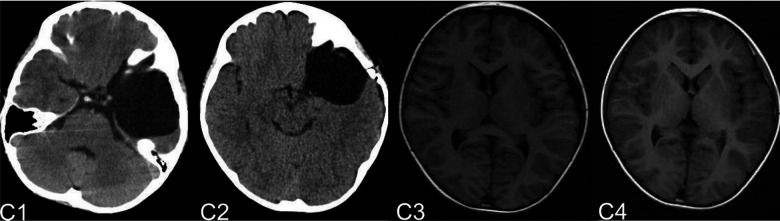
(C) shows the patient, who had subdural effusion after IAC operation during the follow-ups. C1 and C2 shows the CT images before and after operation. C3 shows the subdural effusion 2 months after the operation. C4 shows the recovery of the subdural effusion with the treatment of follow up observation after 5 month.
